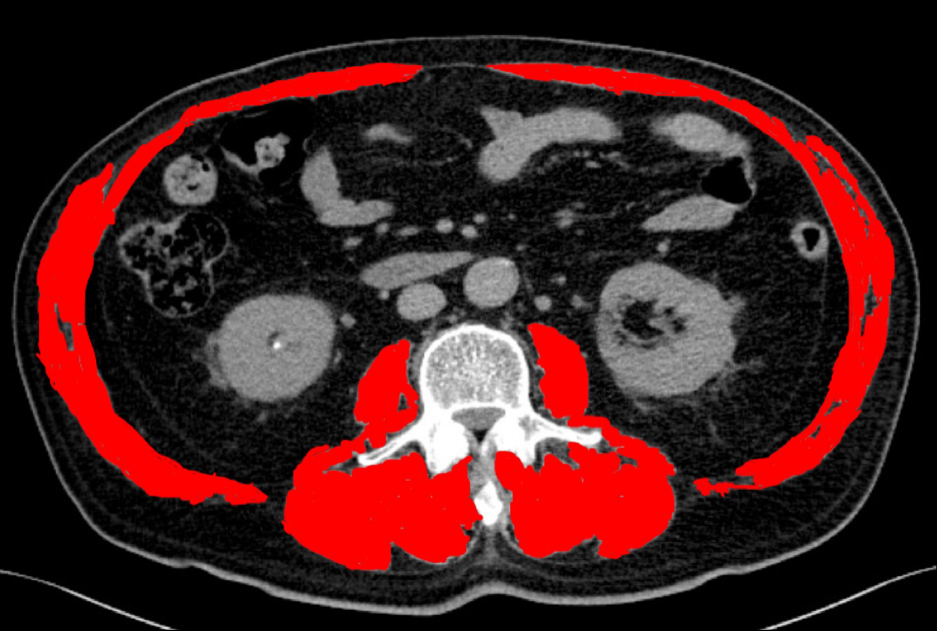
Axial CT Showing SMA at L3. Total area of iliopsoas, erector spinae and abdominal wall muscle are count as SMA (red area).
